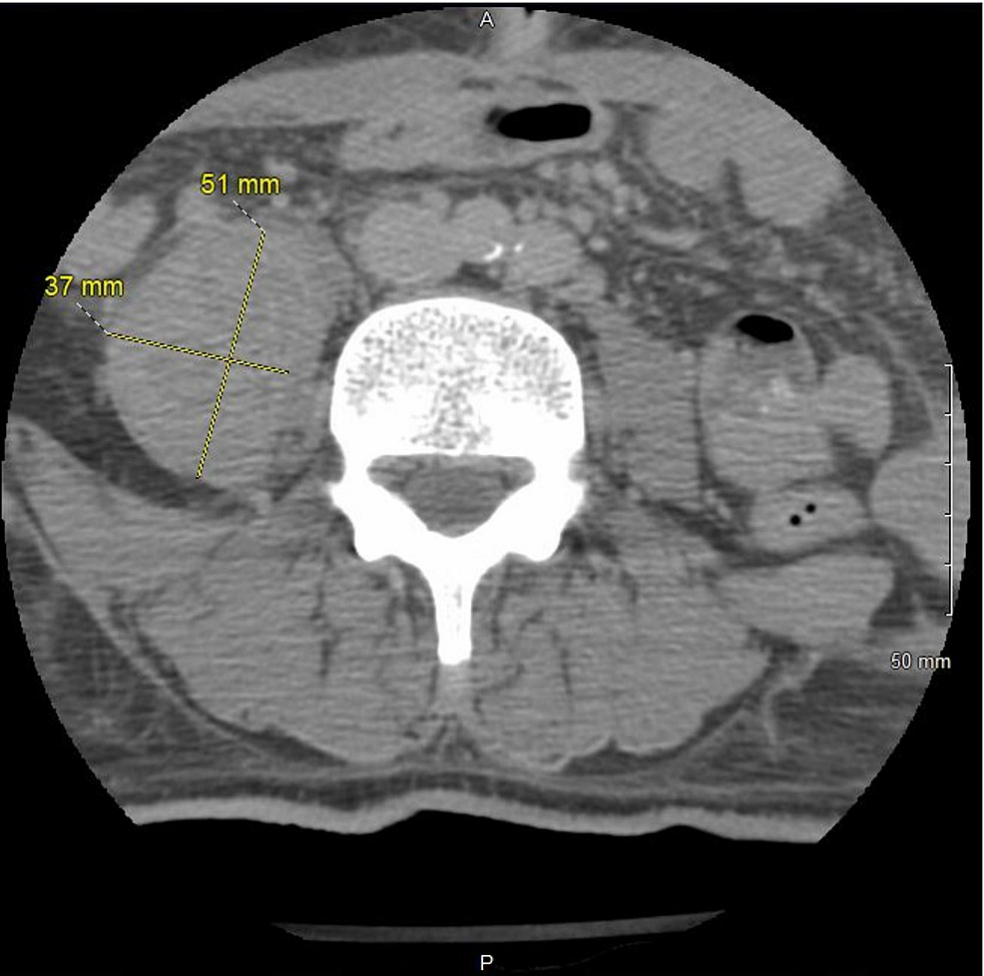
CT spine showing 3.7 cm x 5.1 cm right iliopsoas retroperitoneal hematoma versus abscess
